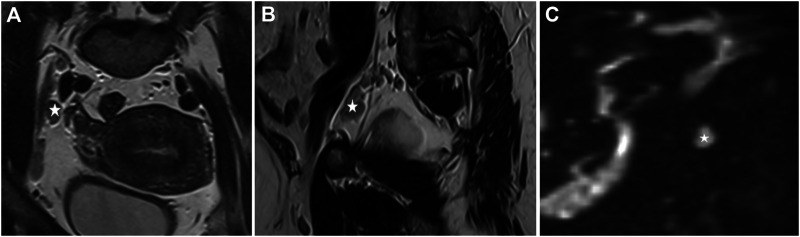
Endometrial cancer, stage T1b. *: Enlarged lymph nodes (A). T2 sequence, coronal view, enlarged lymph nodes near the external iliac artery, (B) T2 sequence, sagittal view, an enlarged lymph node near the external iliac artery, (C) enlarged lymph node, postcontrast DWI (Diffusion-weighted magnetic resonance imaging, b:800).
